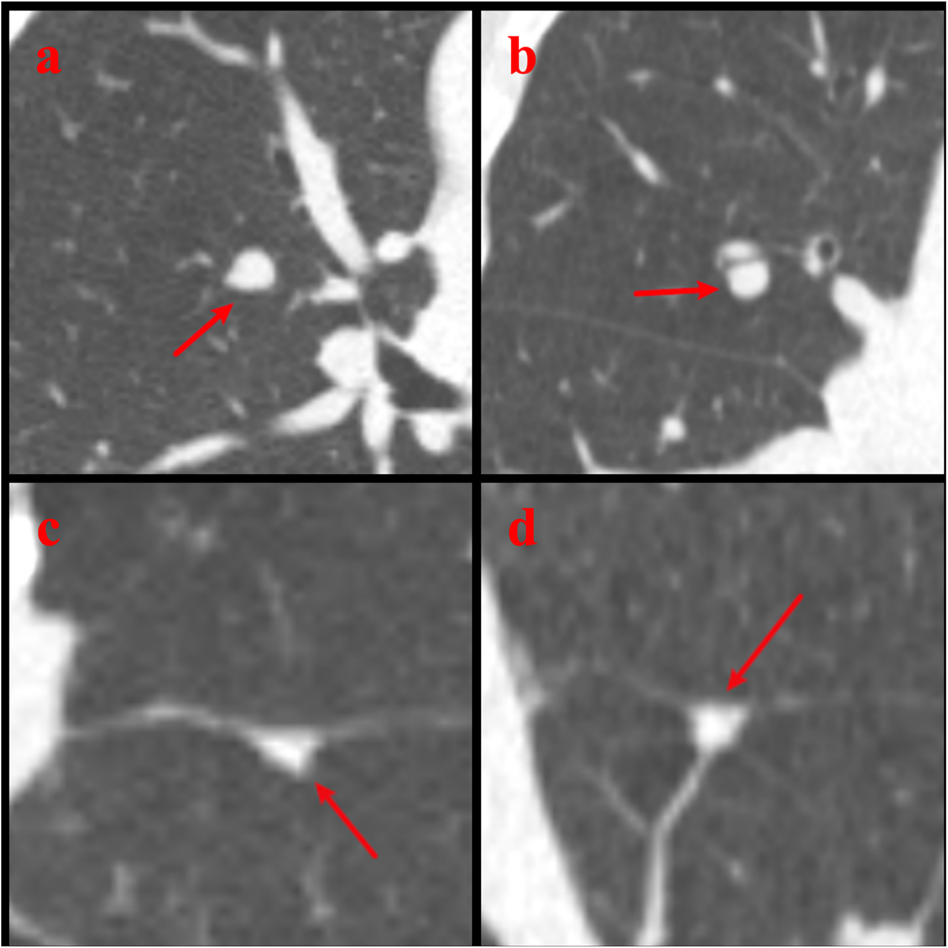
Examples where AI detected the risk-dominant nodule as determined by reference standard. Example 1. LDCT LCS images from a 70-year-old female. a Axial (D45f/1 mm, lung window) and (b) coronal (D45f/1 mm, lung window) LDCT images show a vessel-attached lesion in the right upper lobe (lesion 2). The reference standard determined this lesion as the risk-dominant nodule and classified it as noncalcified solid. AI on all four reconstruction settings detected this nodule and agreed on the nodule type classification with the reference standard. This nodule was the largest nodule for AI-D45f/1 mm, AI-B80f/1 mm, and AI-D45f/2 mm, but not for AI-B30f/2 mm. Example 2. LDCT LCS images from a 69-year-old female. c Axial (D45f/1 mm, lung window) and (d) coronal (D45f/1 mm, lung window) LDCT images show a fissure-attached lesion in the left lower lobe (lesion 1). The reference standard determined this lesion as the risk-dominant nodule and classified it as noncalcified solid. AI on all four reconstruction settings detected this nodule, and this nodule was the largest nodule for AI on all four reconstruction settings. AI-D45f/1 mm and AI-D45f/2 mm agreed on the nodule type classification with the reference standard, while AI-B80f/1 mm misclassified it as calcified, and AI-B30f/2 mm misclassified it as nonsolid. LCS, lung cancer screening; LDCT, low-dose CT
